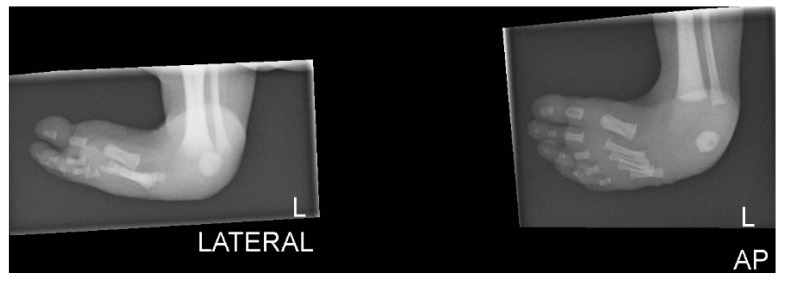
Plain lateral radiograph of foot showing club foot.
